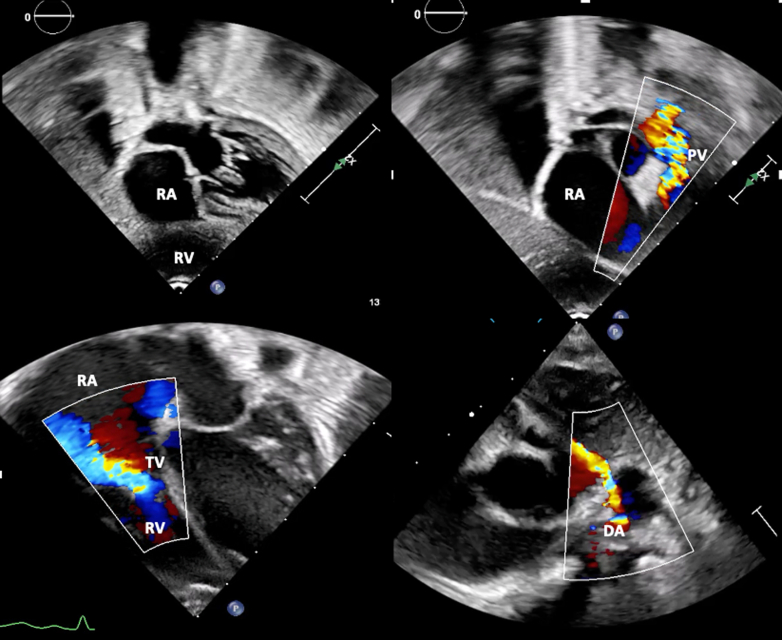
Transthoracic Echocardiography at the Time of Discharge (Top Left) Subcostal 4-chamber view shows a hypoplastic bipartite right ventricle and a dilated right atrium. (Top Right) Color Doppler imaging across the pulmonary valve demonstrates severe stenosis with minimal antegrade flow. (Bottom Left) Color Doppler assessment of the tricuspid valve shows severe to moderate regurgitation. (Bottom Right) Color Doppler of the ductus arteriosus demonstrating shunting. TV = tricuspid valve; other abbreviations as in Figures 1 and 2.
